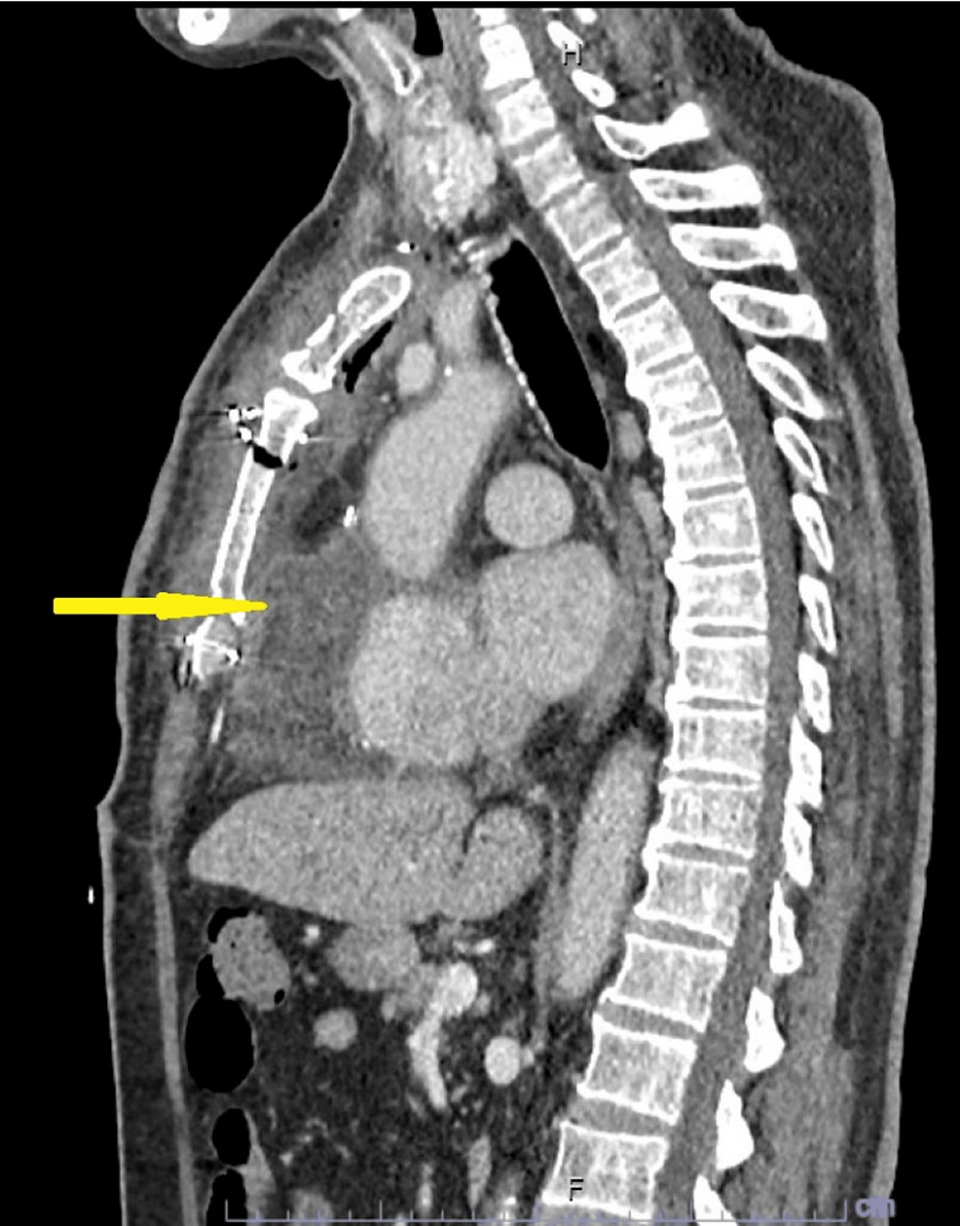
CT scan (coronal view) depicting anterior mediastinal collection (yellow arrow) with air locules and enhancing pericardium, suggestive of infected pericardial effusion.
